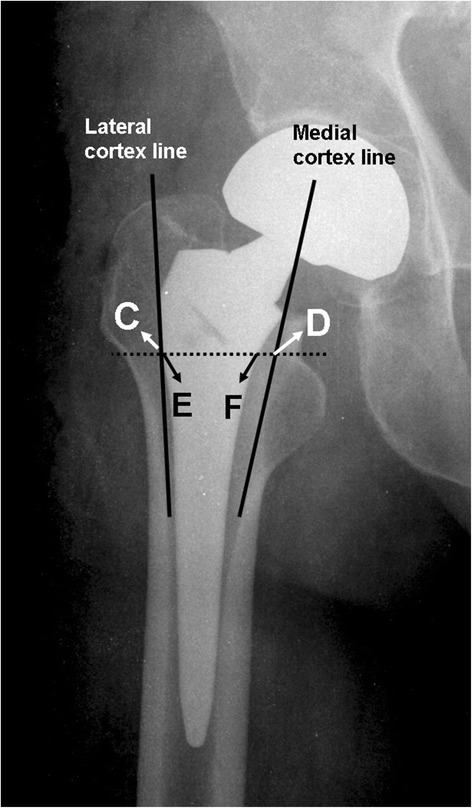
Assessment of the femoral stem-canal ratio. A line was drawn along the lateral margin of the lateral cortex and extended, and then the width between this line and the medial cortex was measured (C-D). Femoral stem-canal ratio was defined as the ratio of the width of the femoral component to the width of the medullary canal (E-F/C-D). Satisfactory was recorded when the ratio was more than 80 %. Radiographic evaluation originally appeared in J Bone Joint Surg Br 1993;75:6-13. E-F: the width of the femoral component
